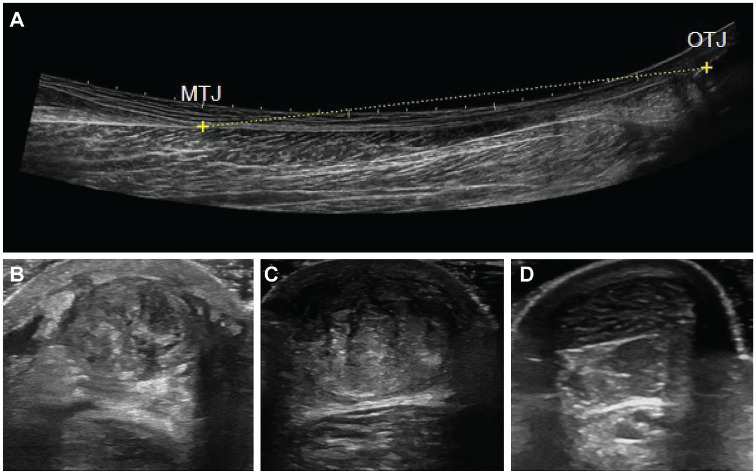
Ultrasound images of Achilles tendon length and cross-sectional area. (A) Length of a healthy Achilles tendon. (B) Cross-sectional area of an injured Achilles tendon at 6 weeks. (C) Cross-sectional area of an injured Achilles tendon at 6 months. (D) Cross-sectional area of a healthy Achilles tendon. MTJ, musculotendinous junction; OTJ, osteotendinous junction.
